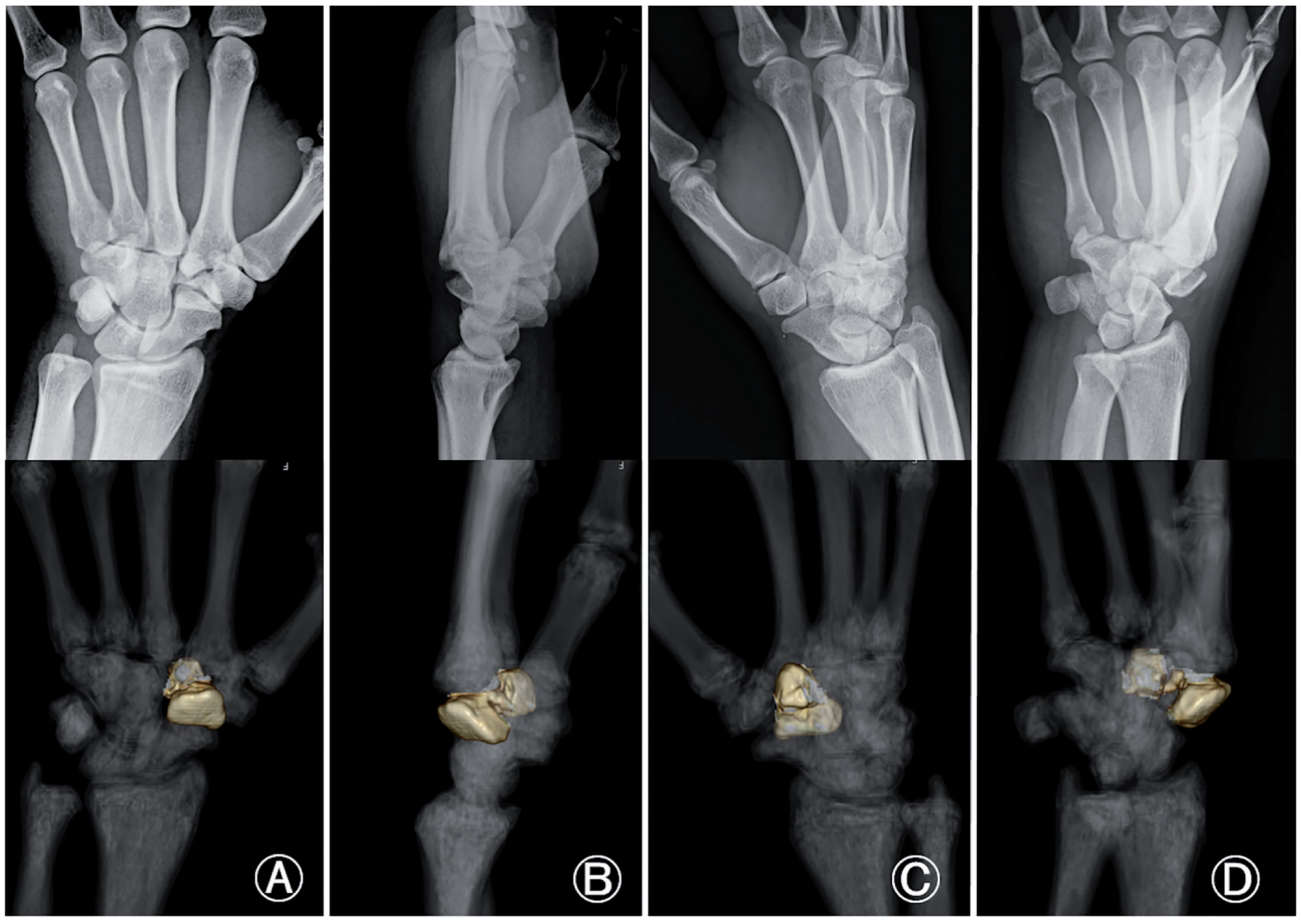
Comparison between X-ray and three-dimensional computed tomography (3 D CT) images of an isolated coronal shearing fracture of the left trapezoid. (A) X-ray in anteroposterior view and the corresponding 3 D CT; (B) X-ray in lateral view and corresponding 3 D CT; (C) X-ray in oblique view in pronation and corresponding 3 D CT; (D) X-ray in oblique view in supination and corresponding 3 D CT. The lateral and the oblique views in supination are important to identify a coronal shearing trapezoid fracture. In particular, the oblique view in supination clearly depicts a coronal shearing trapezoid fracture. No useful information could be obtained from the anteroposterior and oblique views in pronation.
